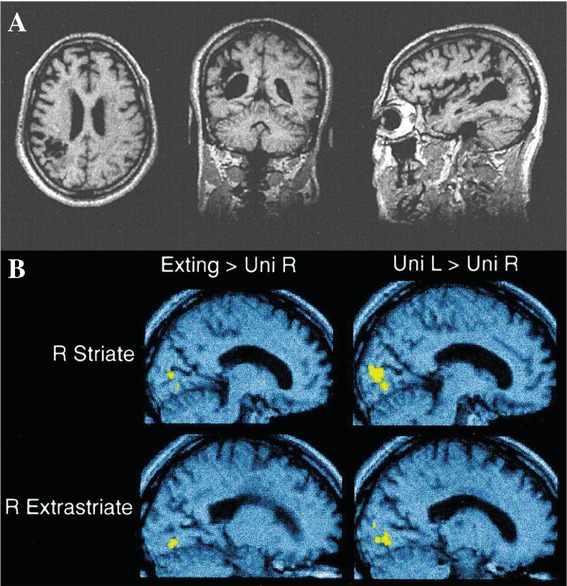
(A) Anatomic location of the lesion causing neglect and extinction in the patient studied in Refs. 15–17. Three sections (axial, coronal, and sagittal) are shown through a T_1_-weighted MRI. A low attenuation area within the right inferior parietal lobule, consistent with an old cerebral infarction, is shown. Note the highly circumscribed nature of the lesion, sparing visual cortex structurally. (B) From Ref. 15, cortical areas activated by consciously seen unilateral stimuli in the left visual field and by extinguished left visual field stimuli on bilateral trials. These panels show the location of the largest and most reliable activations, revealed either by the comparison of bilateral trials (showing extinction) minus unilateral right trials (left panels), or by the comparison of unilateral left trials minus unilateral right trials (right panels). The *x*-coordinate for the slice shown was determined by the peak activation of the comparison it depicts. The upper panels show loci of activation in the striate cortex; the lower panels show those in the early extrastriate area. All activations are superimposed on the T_1_-weighted anatomical image from panel A. The similar location of activations for the two contrasts is evident.
